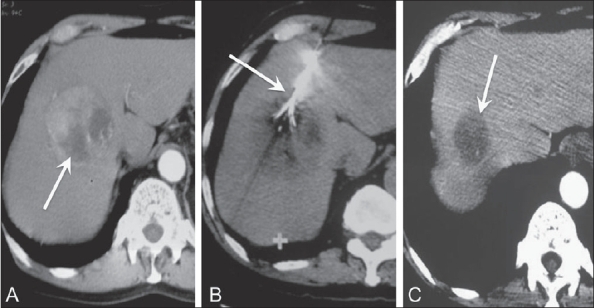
Hepatoma. The pre-ablation axial CT scan (A) shows a heterogeneous mass (arrow). The electrode is seen within the mass (arrow) during ablation (B). The mass shows significant reduction in size with absence of enhancement (arrow) 9 months (C) after the procedurec. Tumor markers: Elevation in organ-specific tumor markers points to recurrence or new lesions.
